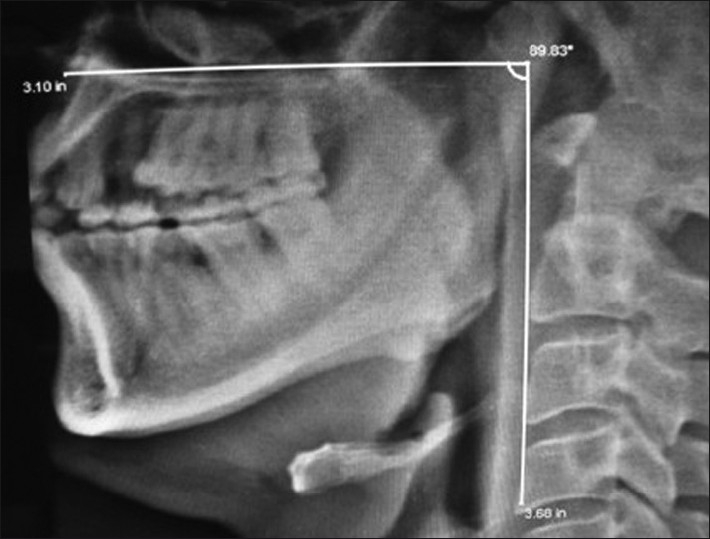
Radiograph of neck - Lateral view showing the abnormal maxillo-pharyngeal angle < than 90 degree (Source: Department of Radio-diagnosis, Imaging and Interventional Radiology N.S.C.B.Subharti Medical College, Meerut)
